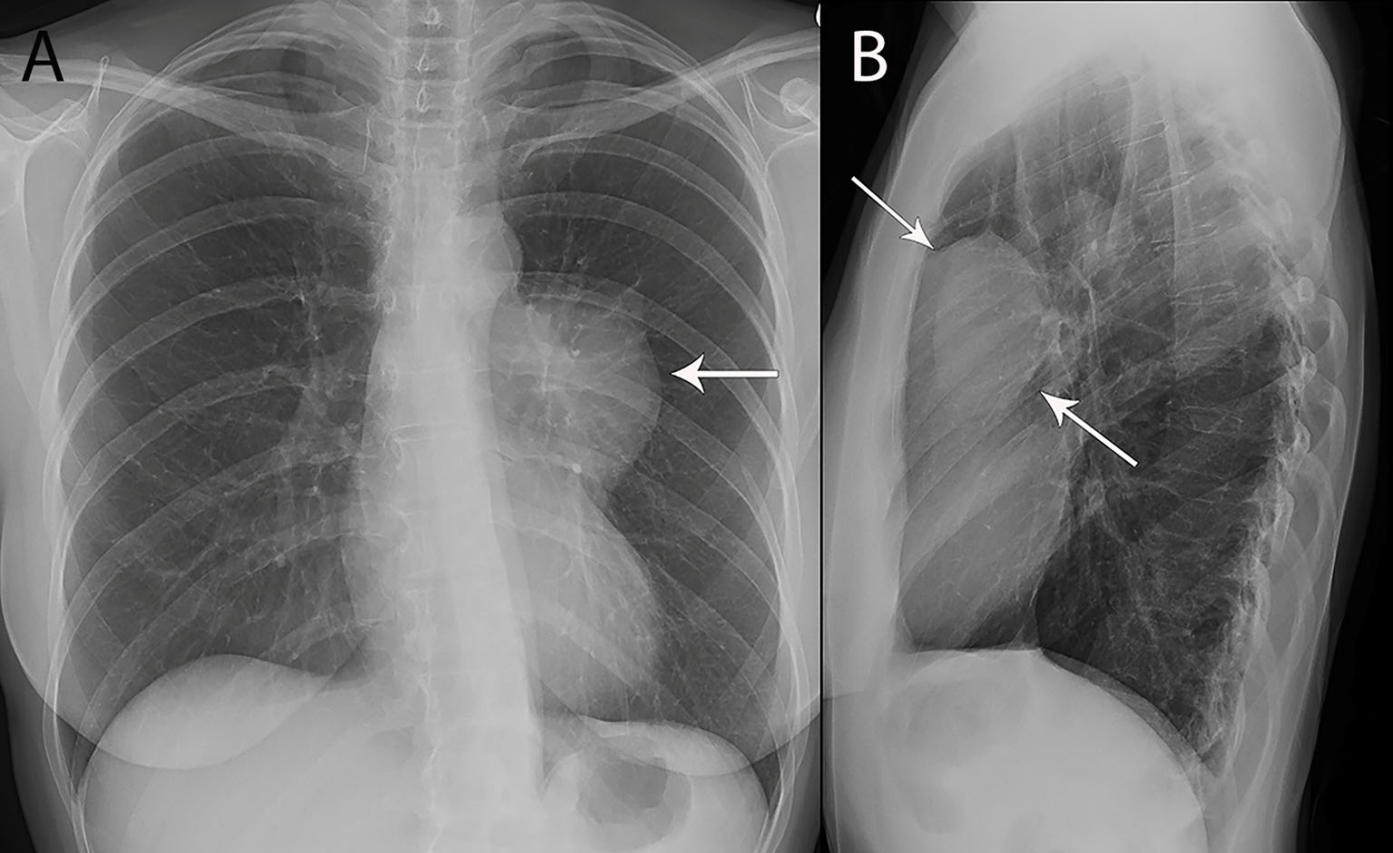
53 year old woman with thymoma. (A, B) Frontal chest radiograph (A) shows left mediastinal contour abnormality (arrow) that results in loss of the silhouette of the upper left heart border. (B) The lesion is localized to the prevascular mediastinum in the retrosternal space (arrows) on the lateral chest radiograph (B).
